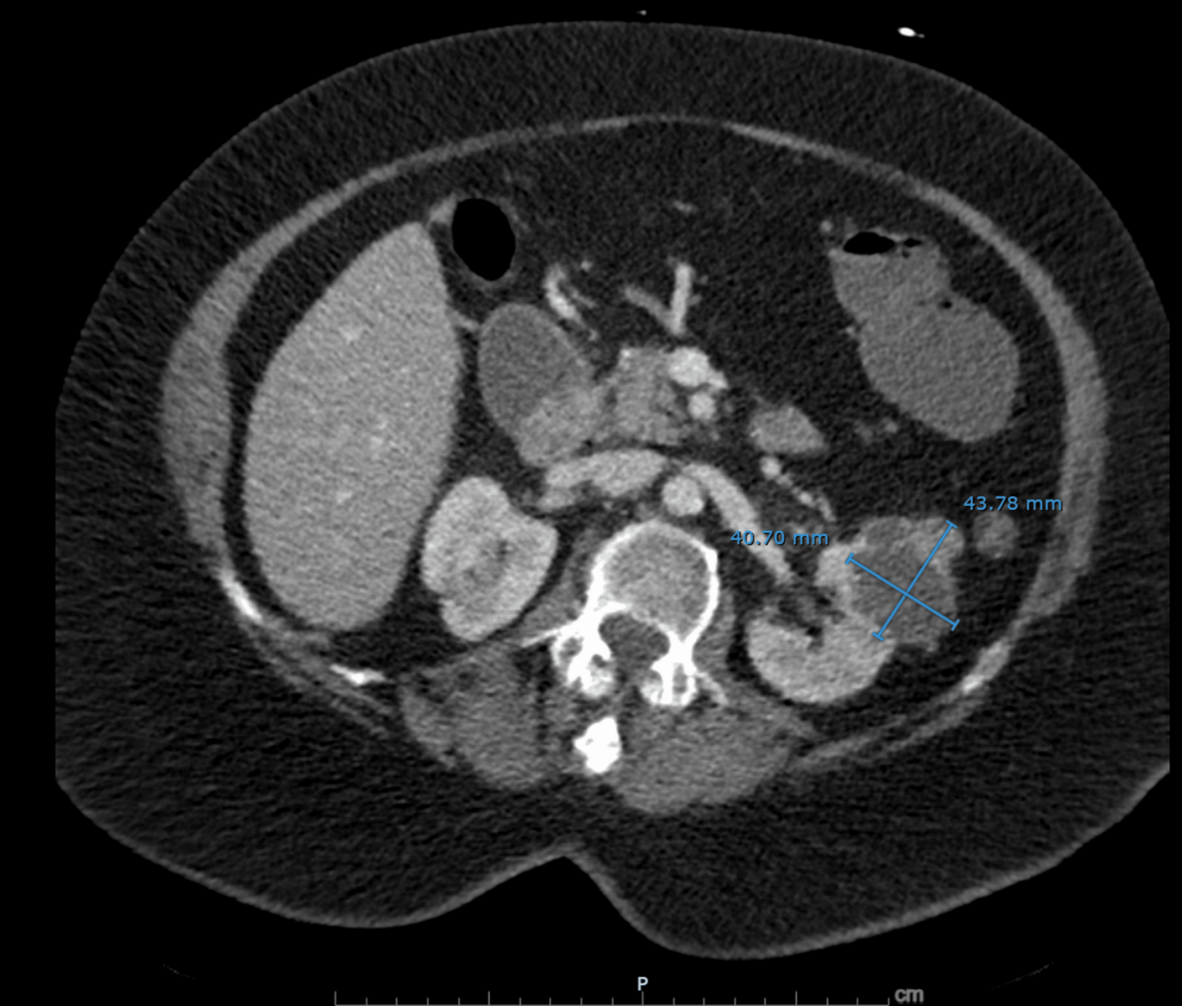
CT demonstrating a left renal mass suspicious for malignancy. Contrast-enhanced computed tomography of the abdomen demonstrates a heterogeneously enhancing exophytic mass in the left kidney measuring 4.4×4.1 cm. The mass does not appear to involve the left renal vein and is highly suspicious for renal cell carcinoma.
